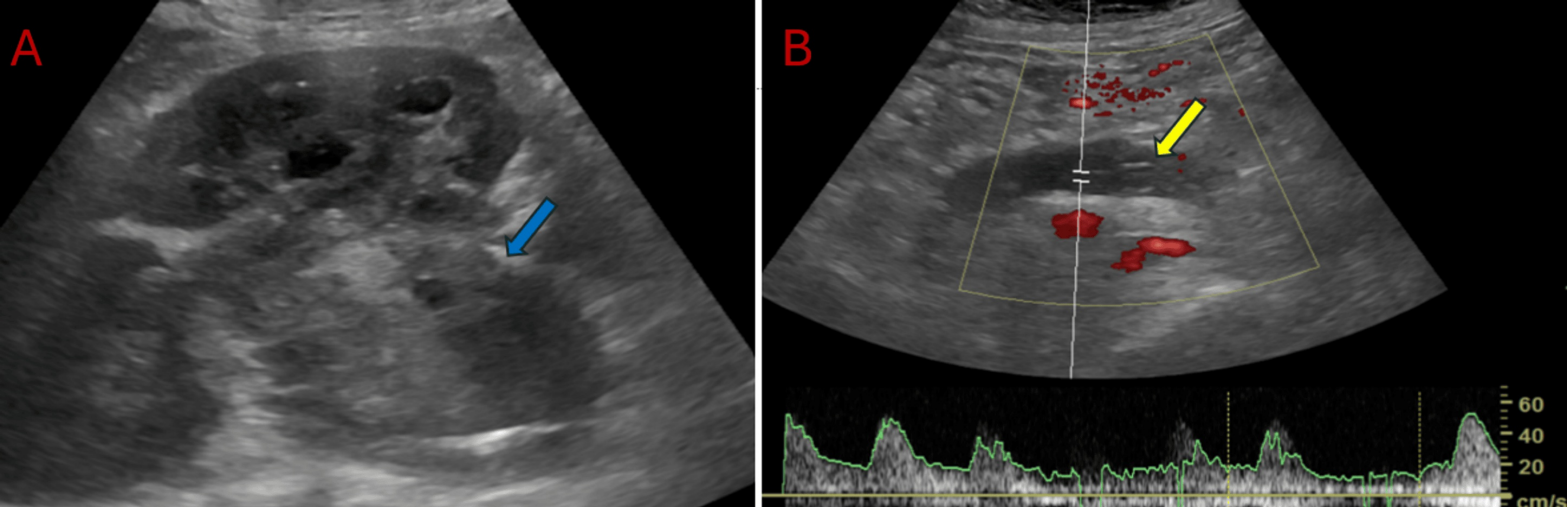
Ultrasound imaging of kidney with Doppler study Ultrasonography images with B mode (A) showing a large exophytic lobulated heteroechoic mass arising from the kidney (blue arrow) and on Power Doppler imaging with spectral waveforms. (B) reveals hypoechoic mass within the left gonadal vein, expanding it with the presence of intermixed arterial and venous waveforms suggesting tumor thrombus (yellow arrow).
